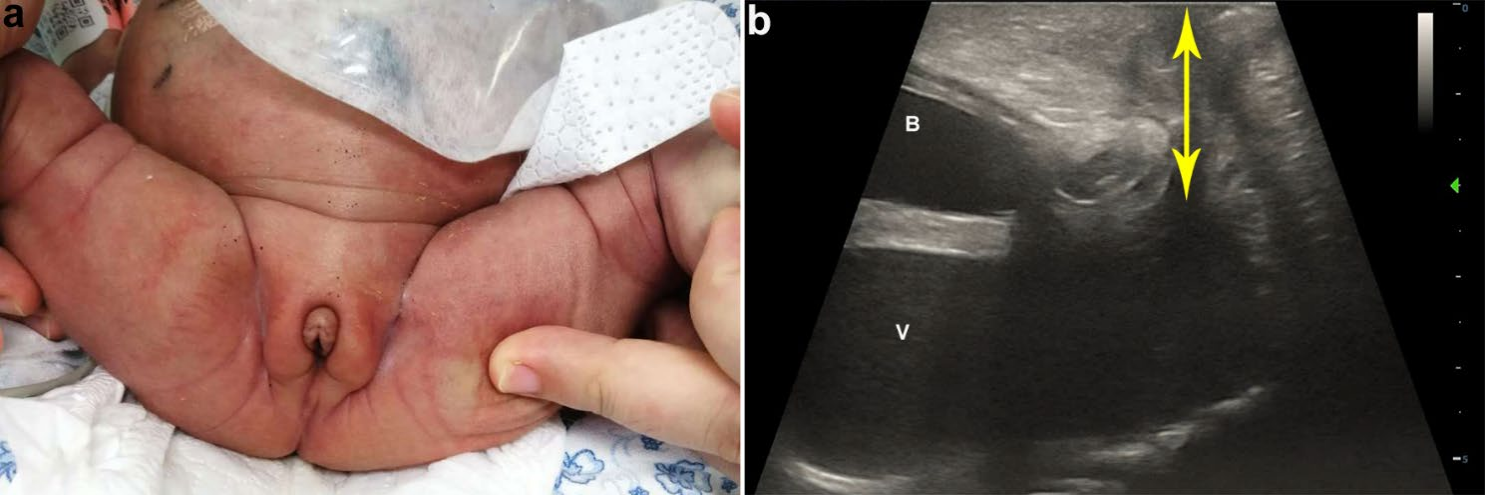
Photo and transperineal ultrasound images of the perineum of newborns. (a) Newborn image shows a single perineal opening where the urethra is expected and the absence of an anal orifice. (b) Sagittal sonogram obtained using a perineal approach on the 34th day after birth. A common canal (double-headed arrow) with a length of about 30 mm. B, bladder; V, vaginal
